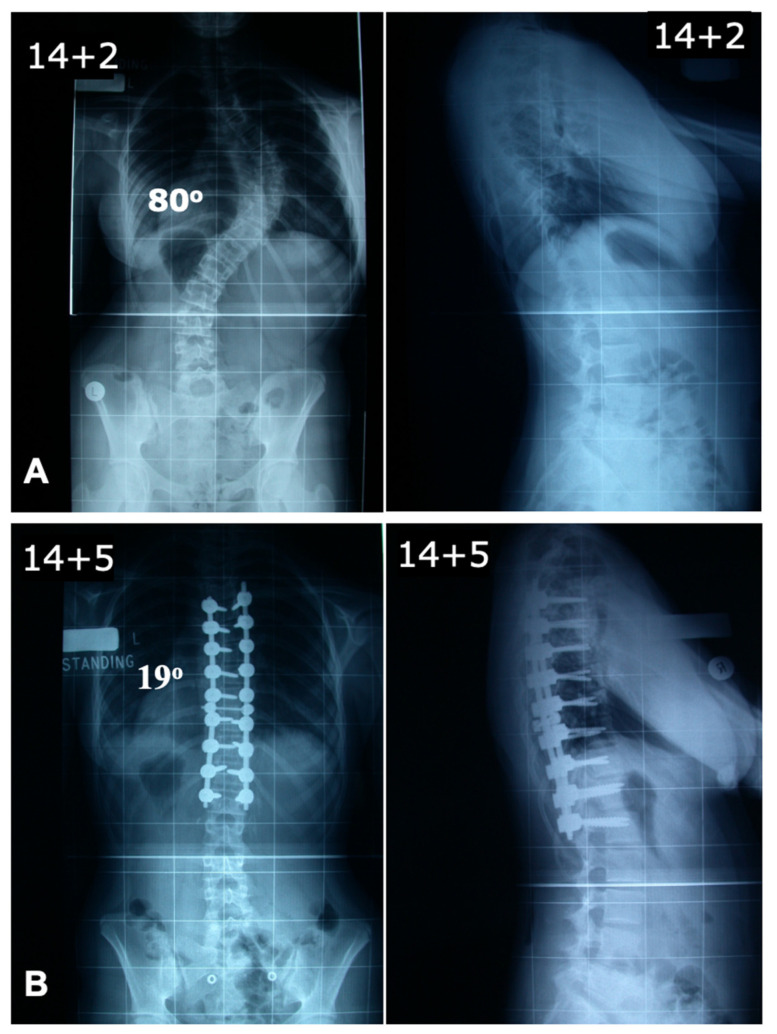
Deformity correction using double-rod technique. (A) Preoperative antero-posterior and lateral radiographs showing a thoracic scoliosis with a Cobb angle of 80 degrees. (B) Postoperative radiographs showing good deformity correction with coronal and sagittal balance.
